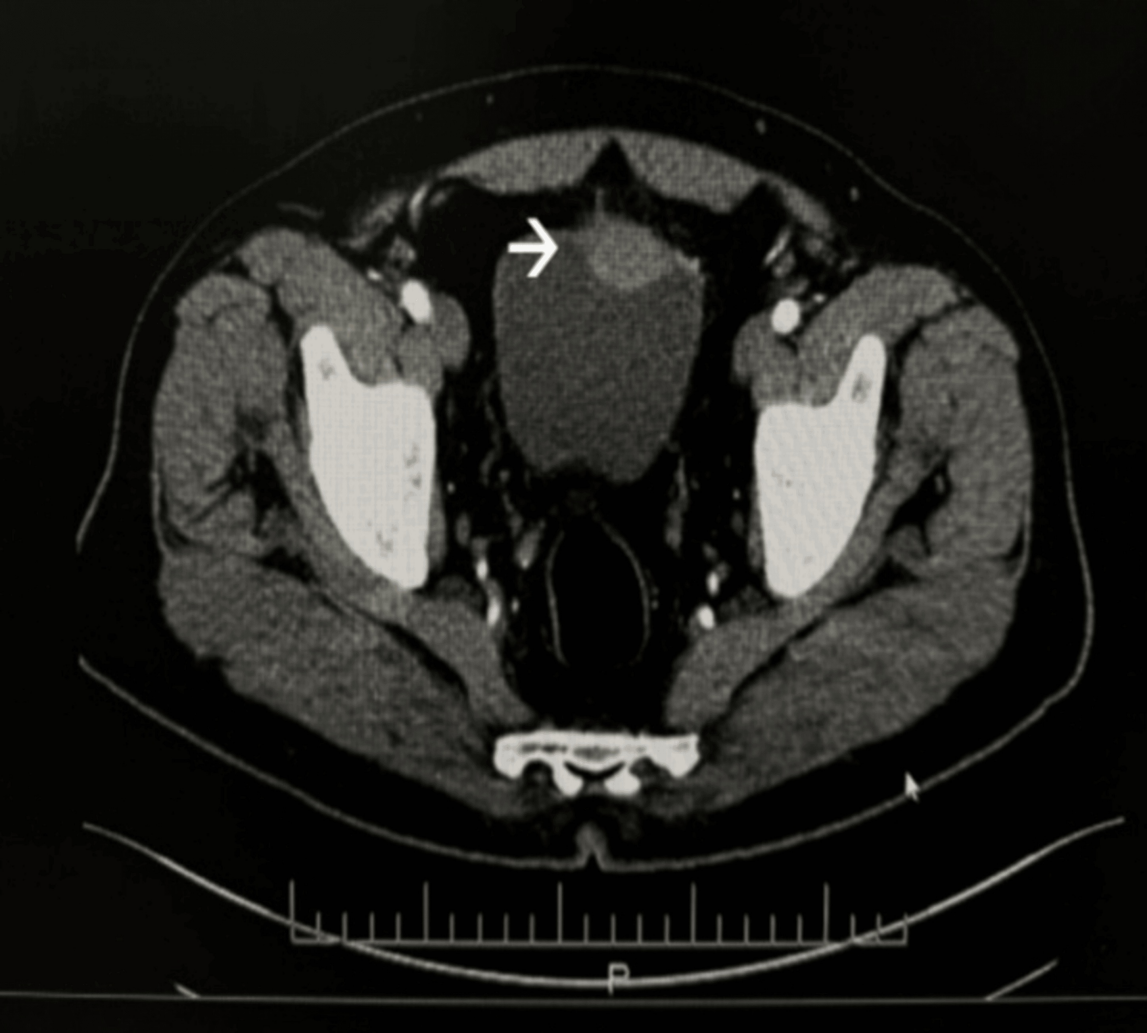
Enhanced CT showing a domal bladder mass (arrowhead).
